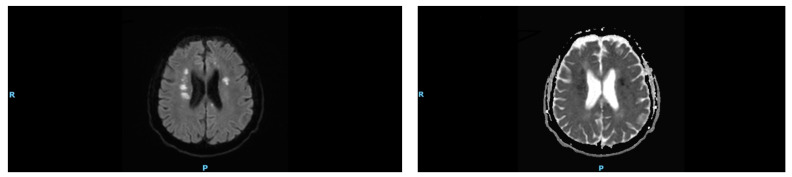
MRI brain showing high signal change in the diffusion-weighted sequence (right), with corresponding hypointensity in the periventricular and left frontal subcortical area in an apparent diffusion coefficient (ADC) map (left).
